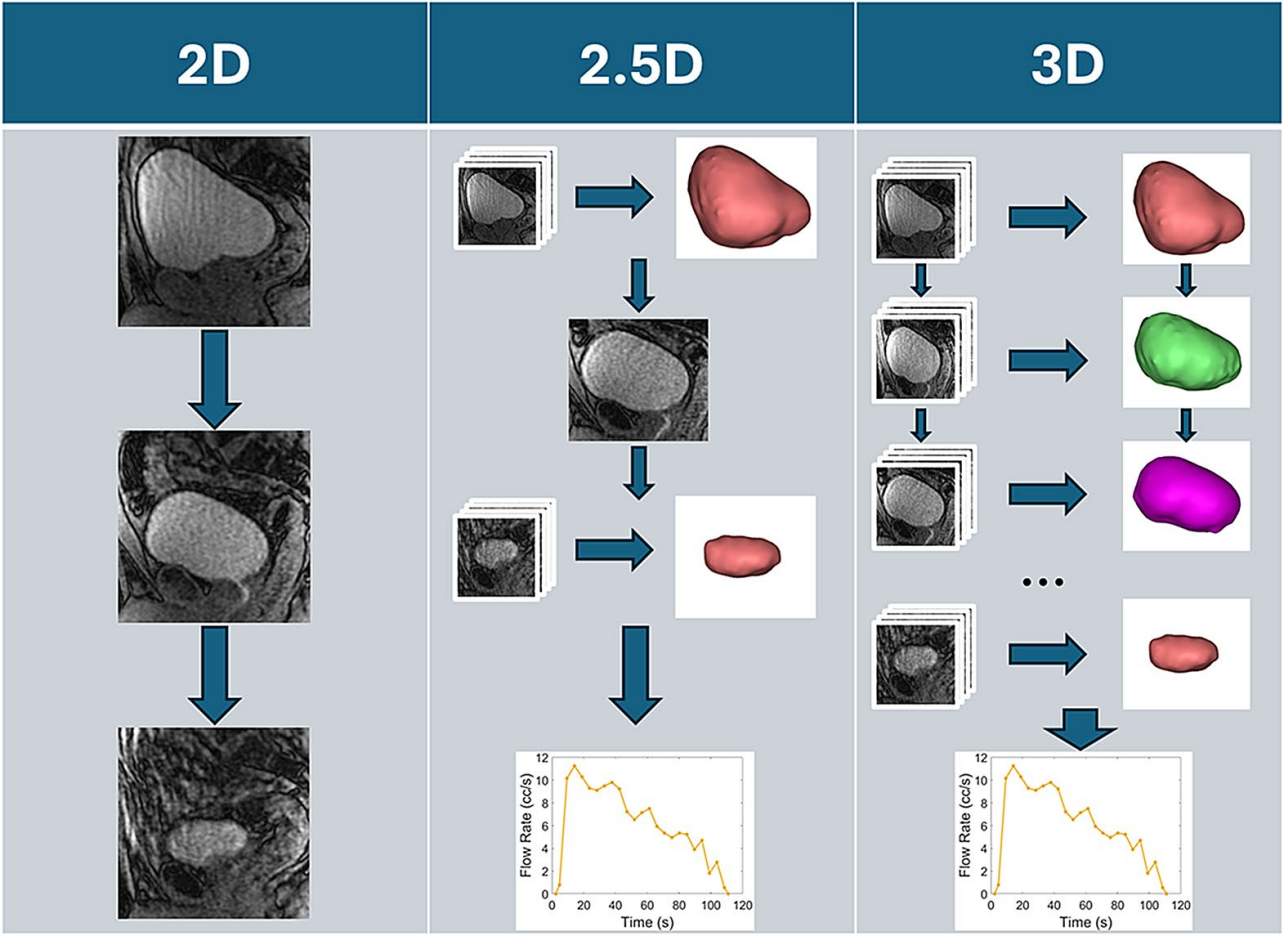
Visual representation of the evolution of dynamic MRI for the evaluation of the LUT, starting with 2D, following with 2.5D and ending with 3D. Initial methodologies use extended acquisition times or real-time acquisition times to quantify in-plane anatomical changes in the LUT. 2.5D methodologies combine volumetric (3D) acquisitions with extended acquisition times with real-time 2D acquisitions to interpolate volumetric changes during diverse processes in the LUT. 3D methodologies use contrast-enhanced volumetric acquisitions with short acquisition times to capture dynamic processes in the LUT such as bladder voiding and filling
